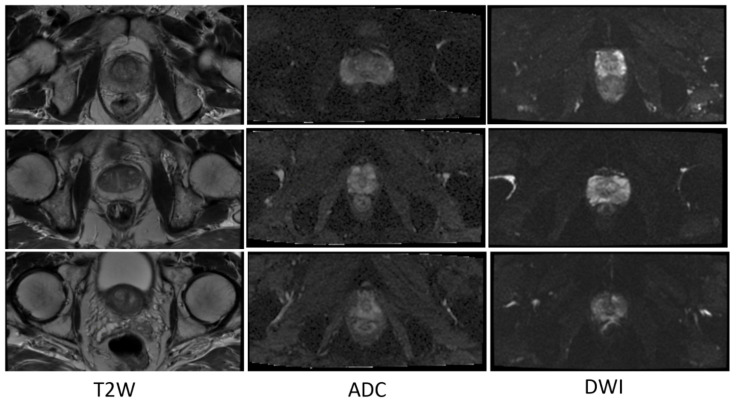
Some examples of T2W, ADC and DWI MR images from our dataset, which demonstrates that DWI and ADC modalities have lower signal around the prostate compared to the T2W modality. Scaled images are shown in Figure A2 for better visualisation.
